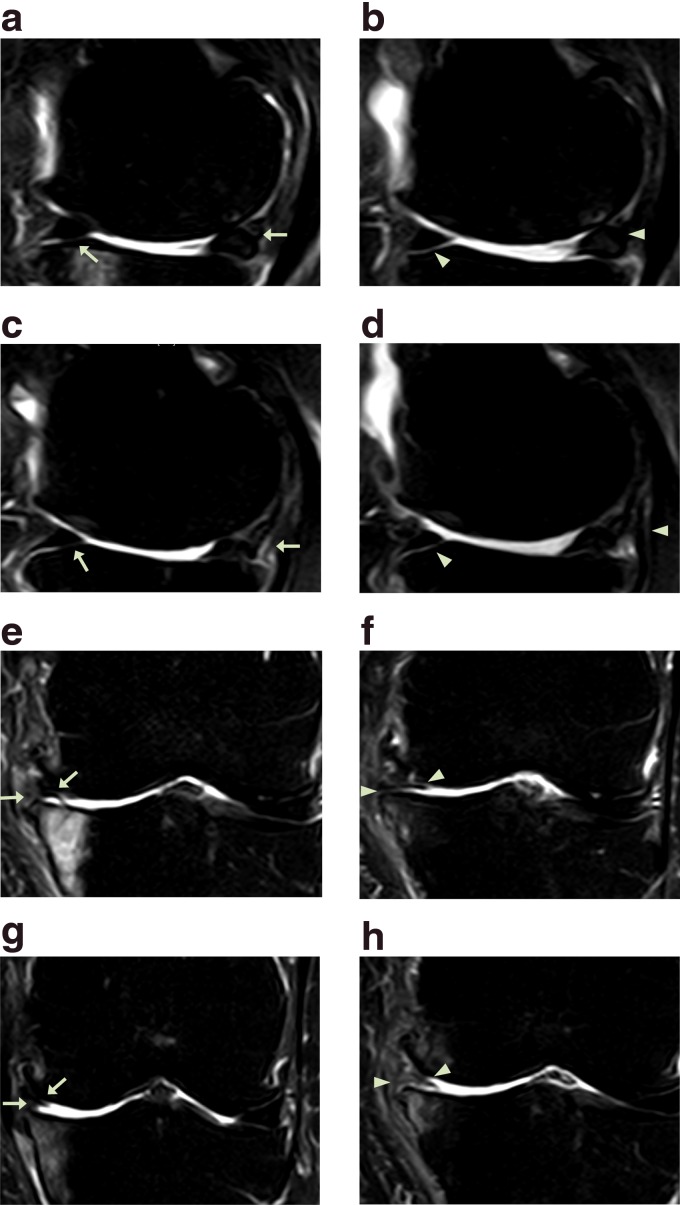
MRI sagittal (a–d) and coronal (e–h) sequential T2 views of the knee from patient #1. Pretreatment MRI scans (a: sequential image, 5/20; c: 6/20; e: 10/20; and g: 11/20) show cartilage lesions (arrows). Post-treatment MRI scans at 16 weeks (b: 6/20; d: 7/20; f: 10/20; and h: 11/20) indicate cartilage-like tissue regeneration (arrowhead) that has been repaired by ADSC/ECM mixture-based treatment. ADSC, adipose tissue-derived stem cell; MRI, magnetic resonance imaging.
